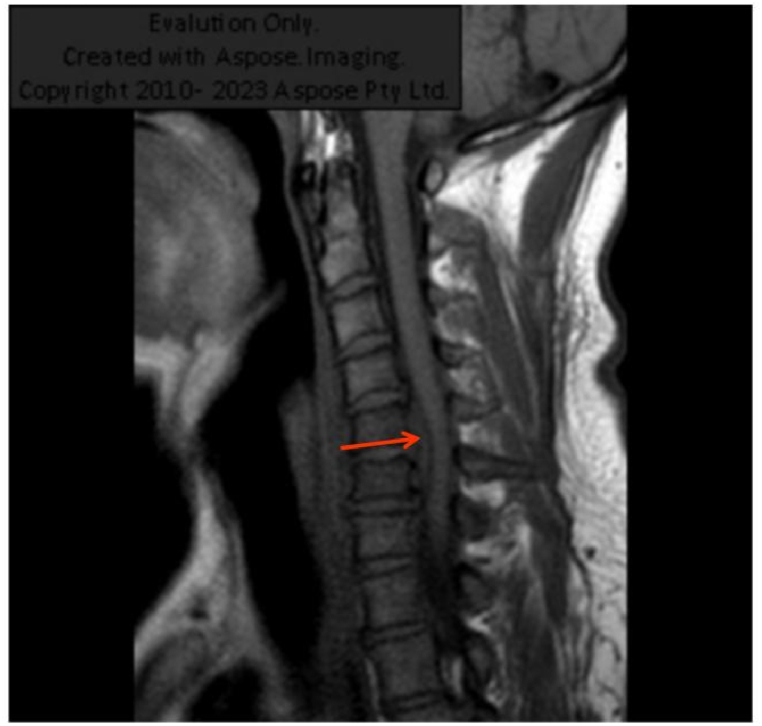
Neck magnetic resonance image showing an abscess.
